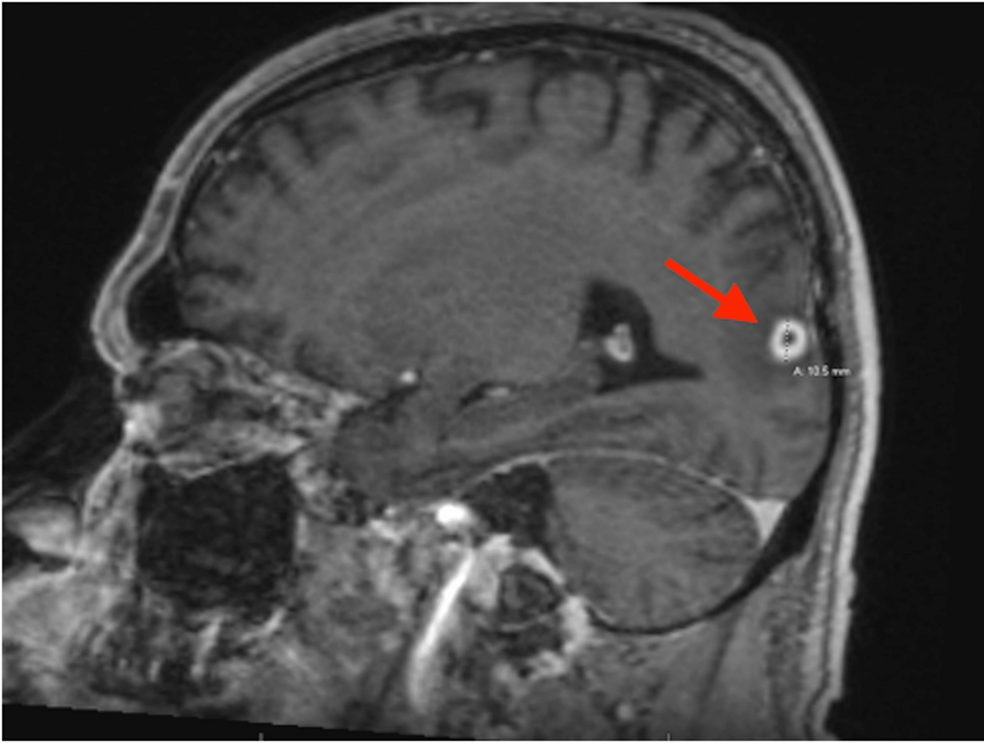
MRI of the brain with 10.5mm lesion in parieto-occipital lobe (FLAIR, sagittal view)
